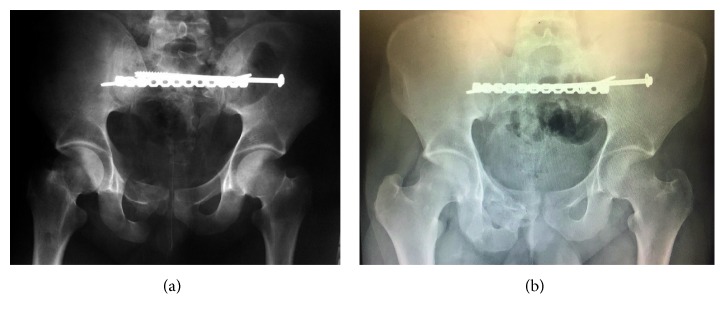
One-month (a) and one-year (b) postoperative AP radiographs.
